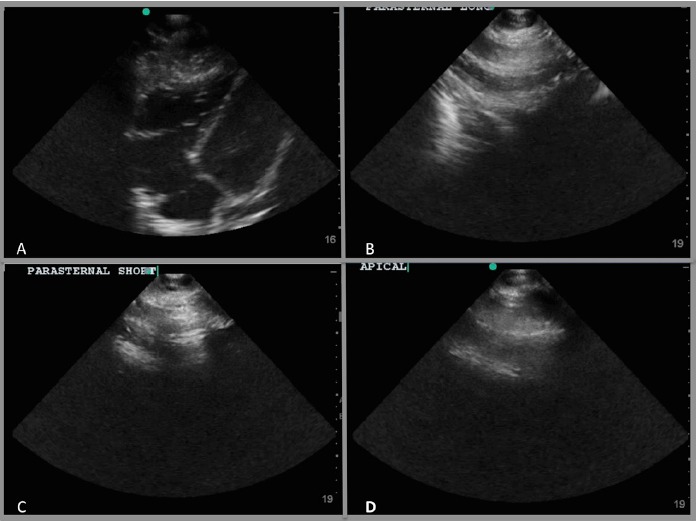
Bedside ultrasound images of patient with pneumomediastinum. (A) The subxiphoid view demonstrated normal cardiac contractility, normal chamber size, and lack of pericardial effusion. The parasternal long (B), parasternal short (C), and apical views of the heart were of poor quality with diffuse A lines (D), suggesting air artifact. These findings were suggestive of the presence of a pneumomediastinum.
